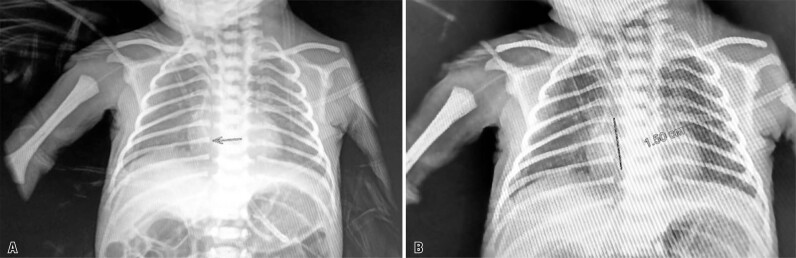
Radiographs showing the peripherally inserted central catheter shortly after insertion: A) Red arrow shows the intracardiac location of the catheter tip; B) Red line shows the extent of catheter retraction required (1.5 cm)
